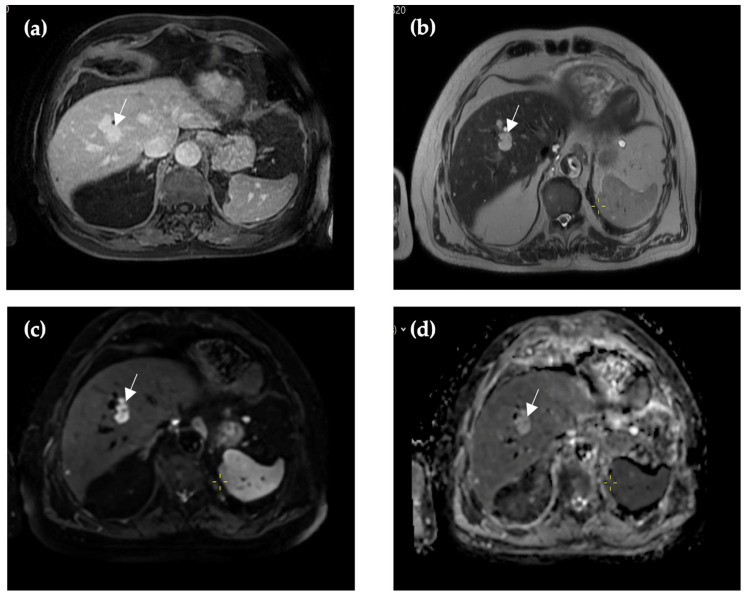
Sixty-four months after first imaging, CE-MRI shows liver lesions (arrow) without negative dynamics in T1 (a), T2 (b), DWI (c), ADC (d) sequences. Hyperintense signal in ADC and limited diffusion in high b-values in DWI refers to the possibility of a benign lesion.
